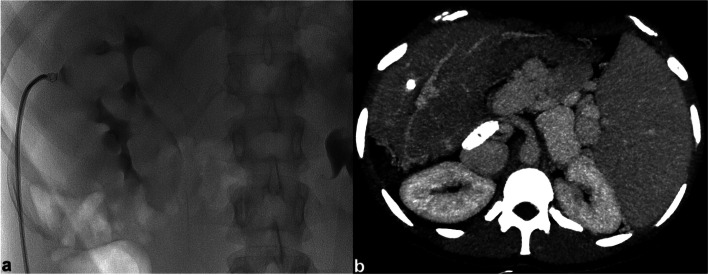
Embolisation of transhepatic tract with micro-coil after PPVC. Fluoroscopy image shows the deployment of a metallic micro-coil in the hepatic parenchyma tract, through a 4F catheter during its retraction (a). MIP reconstruction of contrast-enhanced CT performed after the procedure displays the location of micro-coil in the hepatic parenchyma and confirms the segmental portal branches patency (b)
